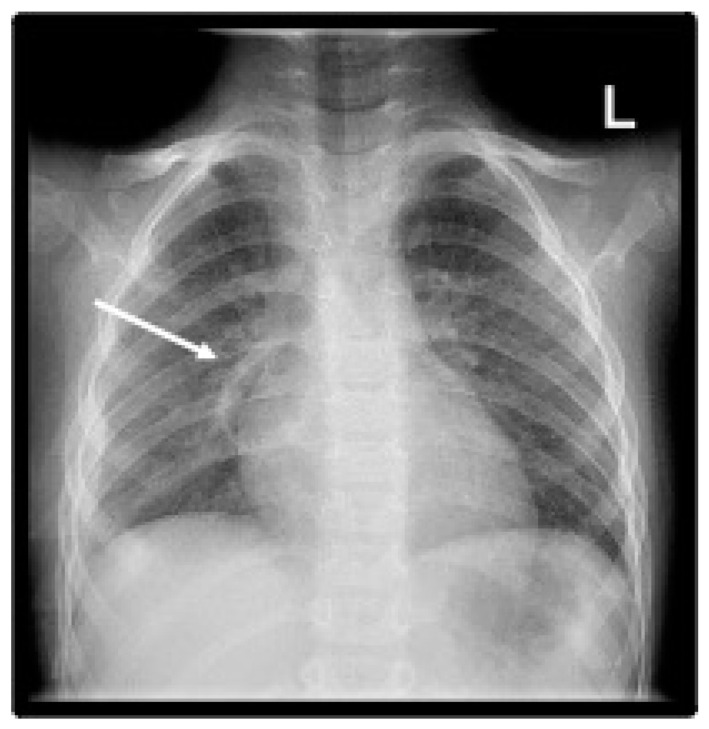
Day 20 of hospital admission. Frontal chest X-ray reveals right paracardiac thin-walled postpneumonic pneumatocele (white arrow).
